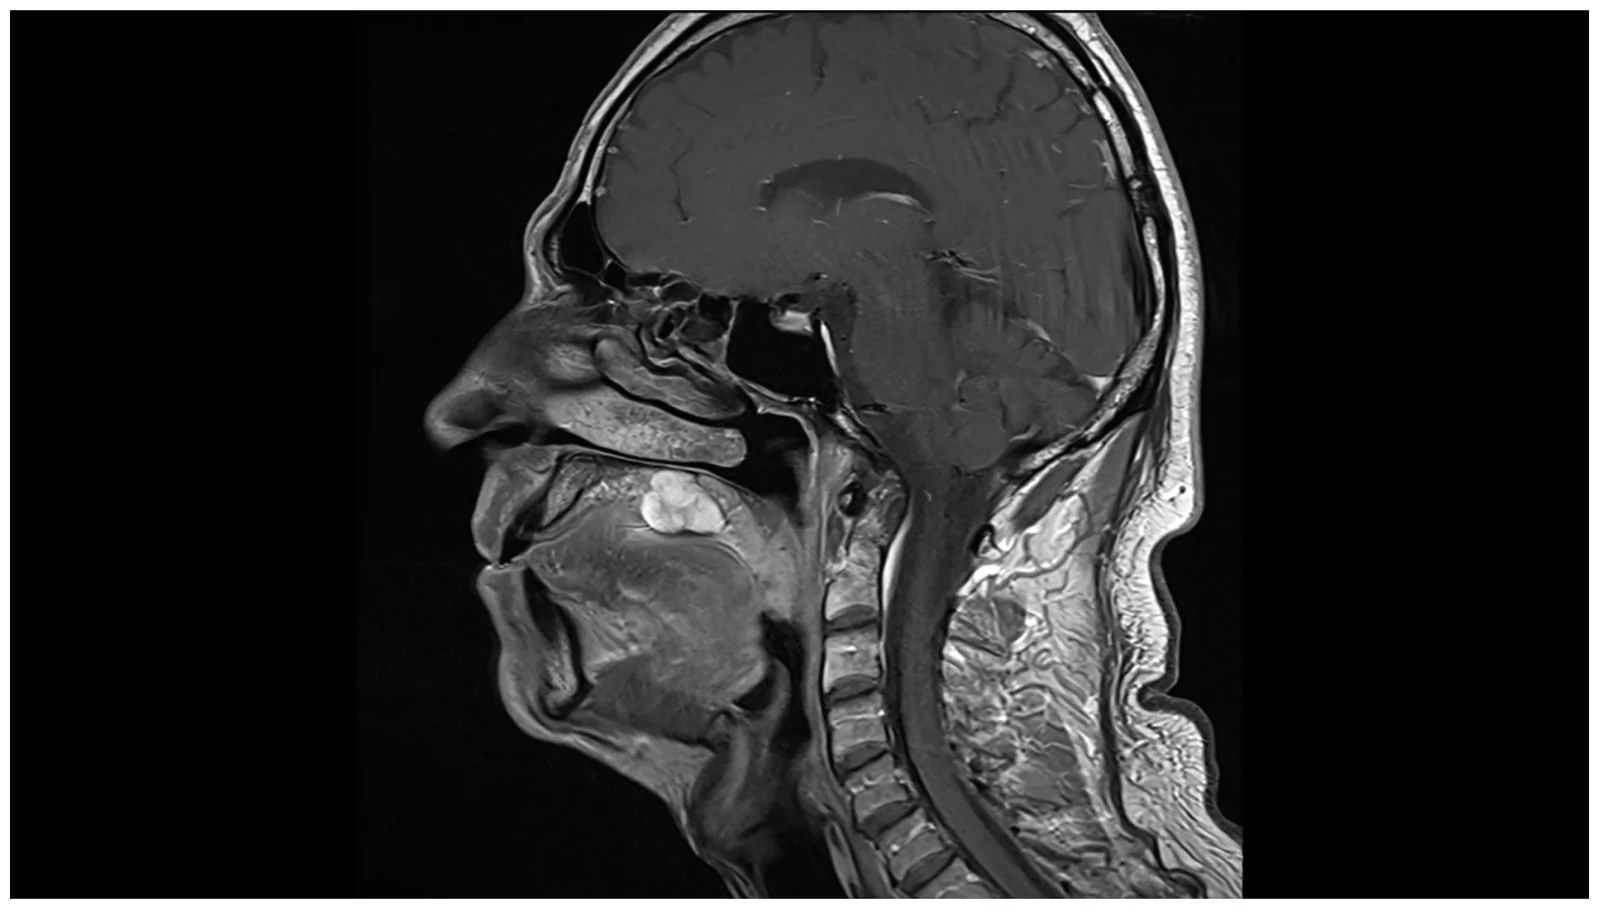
Sagittal contrast-enhanced T1-weighted image demonstrates marked heterogeneous enhancement of the palatal lesion.
